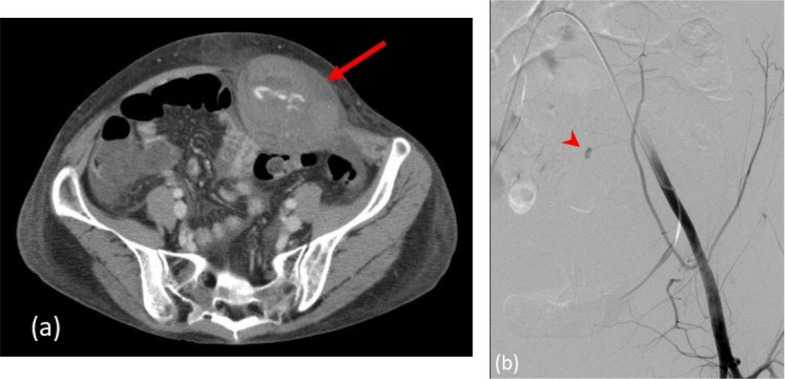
Spontaneous rectus sheath hematoma in a 37-year-old woman with cirrhosis. a Contrast-enhanced computed tomography demonstrated an abdominal wall hematoma with active contrast extravasation (arrow). b Subsequent angiography shows contrast extravasation from a branch of the left inferior epigastric artery (arrowhead). Transcatheter arterial embolization was performed using coils
